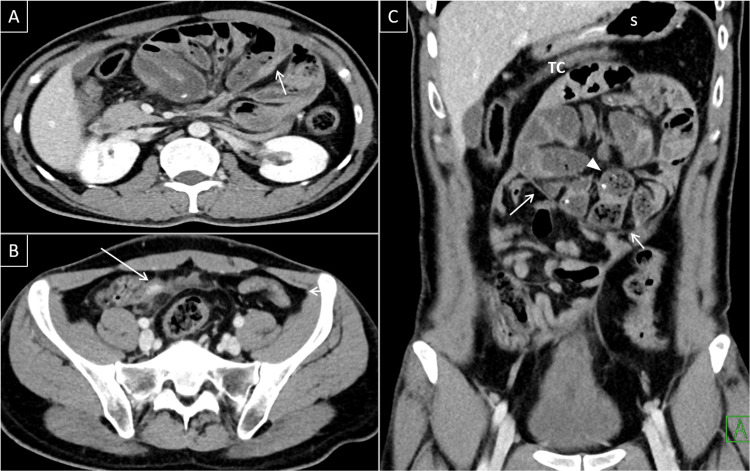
Abdominal CT images. Axial (A and B) and coronal (C) contrast-enhanced CT images showing a cluster of dilated small bowel loops that are drawn into the center of the abdominal cavity, pushing the stomach (S) and transverse colon (TC) superiorly. Note enhanced thickened membrane surrounding the bowel loops (arrows) and the fecalization of small bowel content (arrowhead in C). Thickened wall appendix with surrounding fat stranding (long arrow in B).
